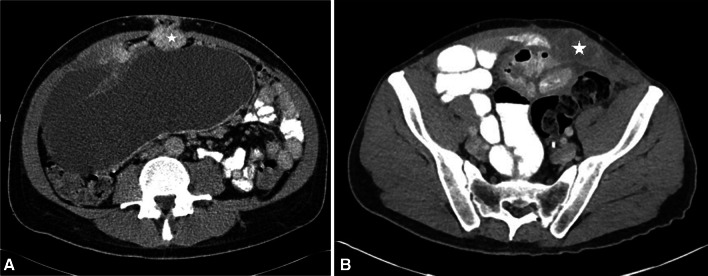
a, b: Abdominal wall disease in two different patients with malignant peritoneal mesothelioma. a CT of a patient with sarcomatoid type of peritoneal mesothelioma showing enhancing soft tissue nodule (*) in the abdominal wall. b CT of a patient with epithelioid mesothelioma shows a large lateral abdominal wall mass (*) abutting and tethering small bowel loop
